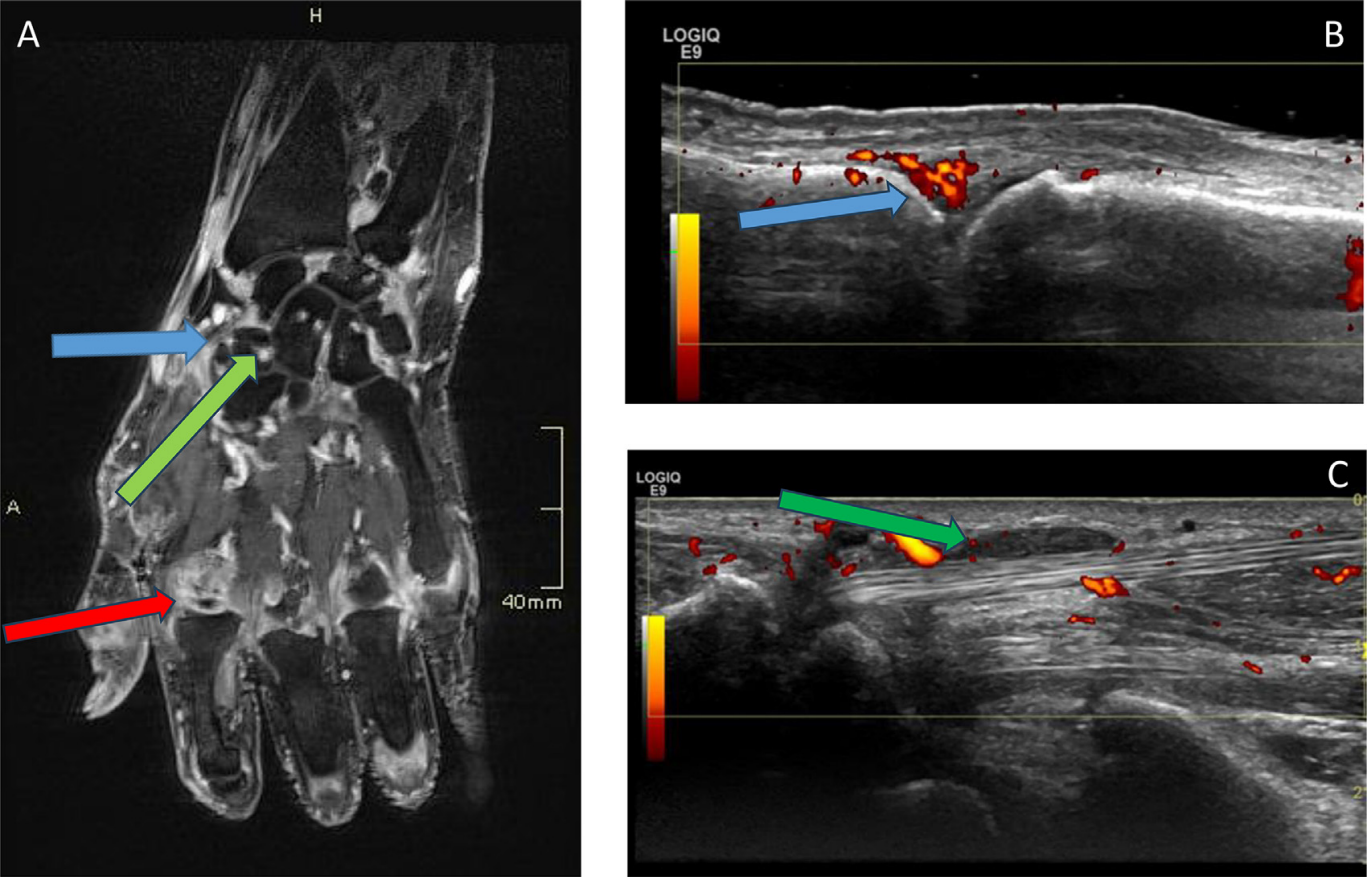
Examples of imaging in patients with ICI-IA. A, 3T MRI scan of hand and wrist using gadolinium contrast. Arrows show extensive synovitis at the wrist and metacarpophalangeal joints with erosions (blue, red and green arrows respectively). B, ultrasound of metacarpophalangeal joint with grade 3 power Doppler signal and isoechoic synovitis (blue arrow). C, Ultrasound showing tenosynovitis of flexor carpi radialis (green arrow). Images courtesy of Dr Mankia.
